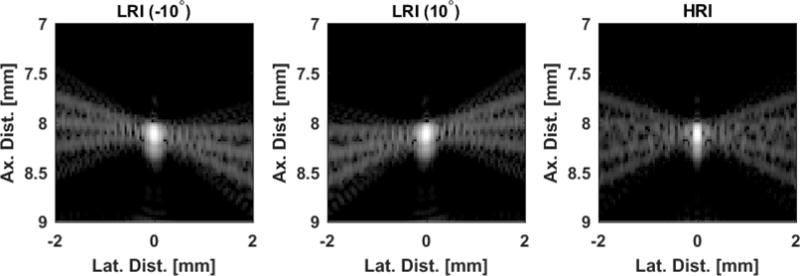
The coherent compounding approach generates high-resolution images (HRIs)by summing together low-resolution images (LRIs) formed at different steering angles. If we look at each LRI individually, we can see that the point spread function (PSF) rotates about the center as the steering angle changes. If we use the LRIs to produce Doppler estimates, the sidelobe rotation could be misinterpreted as tissue motion by the lag-one autocorrelation algorithm. Images are rendered at a 60-dB scale and are normalized to their corresponding maxima.
